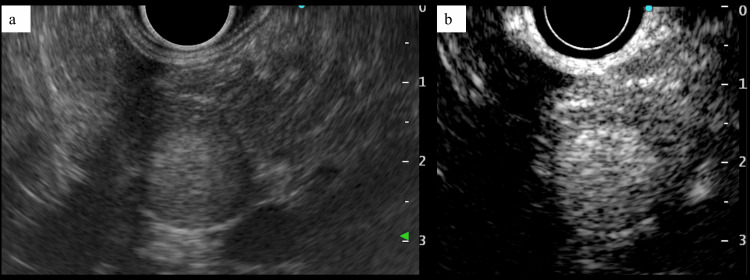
EUS and contrast-enhanced EUS (a) EUS shows a 12-mm mass in the pancreatic tail with higher echogenicity compared to the surrounding parenchyma. (b) Contrast-enhanced EUS reveals a homogeneous, hypervascular lesion in the pancreatic tail, consistent with mRCC, which typically presents as a hypervascular mass. EUS, endoscopic ultrasonography; mRCC, metastatic RCC
